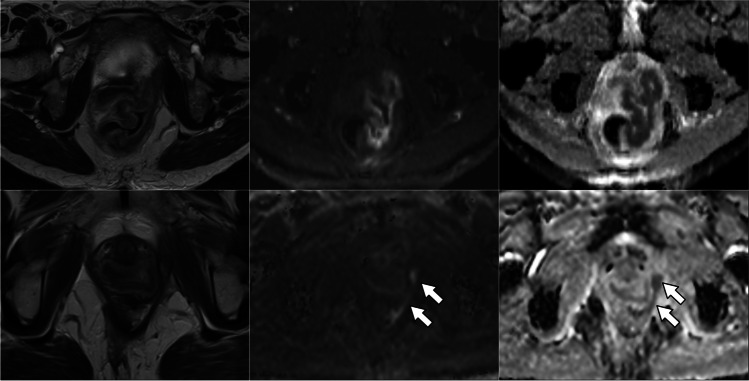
Images in a 41-year-old woman with squamous anal carcinoma. T2-weighted MRI (right column), high *b*-value DWI (middle column) and DWI ADC map (left column). At baseline MRI, tumour staged as T4 invades the vagina posteriorly and left pelvic sidewall anteriorly (upper row). After treatment (lower row), the presence of both linear and nodular diffusion restriction within a cavity left by tumour shrinkage, not spatially matched to anorectal mucosa (arrows), was deemed indeterminate (TRG-3 and DWI-TRG-3) by most observers. Complete response was recorded clinically
